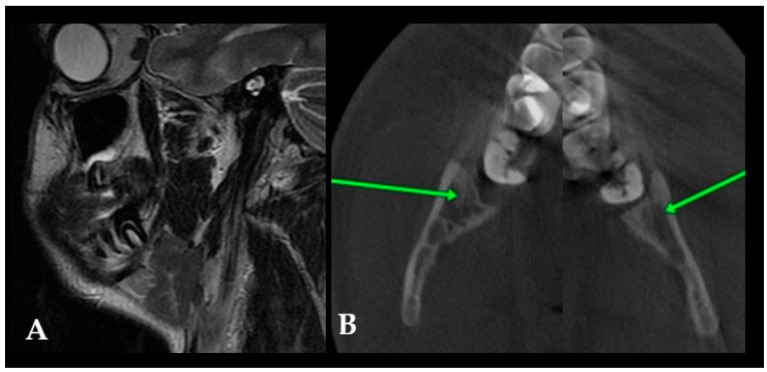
Postoperative magnetic resonance ((A), sagittal view) and CBCT (B) were conducted to assess bone healing. Green arrows ((B), axial view) point to good bone healing and proper bone structure after the procedure.
